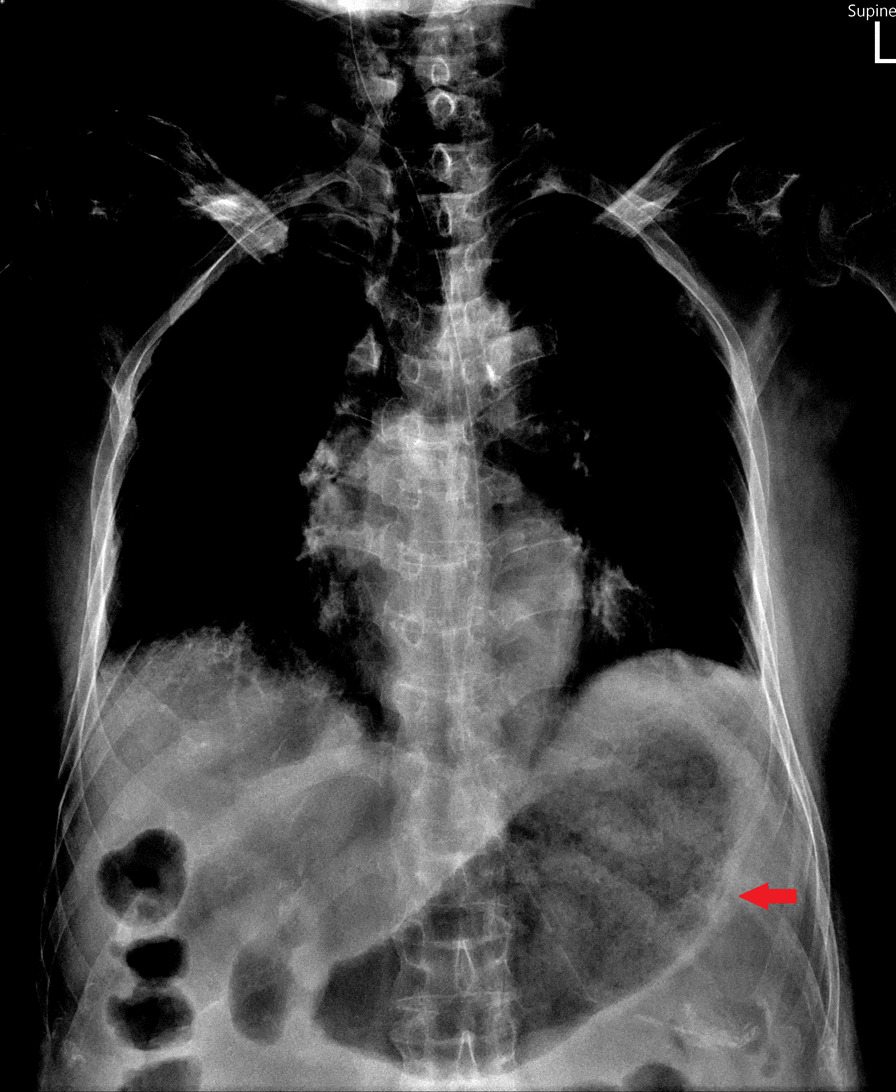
The chest X-ray showed distended stomach with thickened and blurred margin of the gastric fundus
